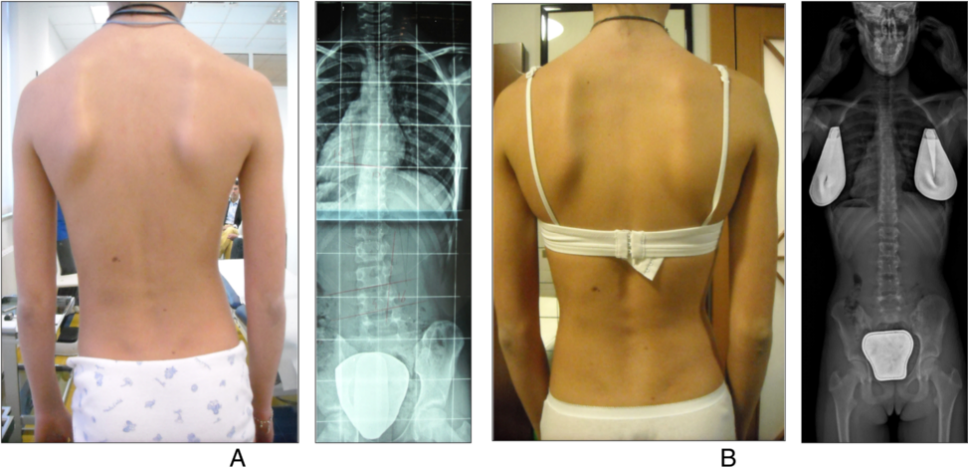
Photograph and radiograph of a patient with scoliosis before SEAS (a) and 43 months later after 3.5 years of SEAS without bracing (b)
